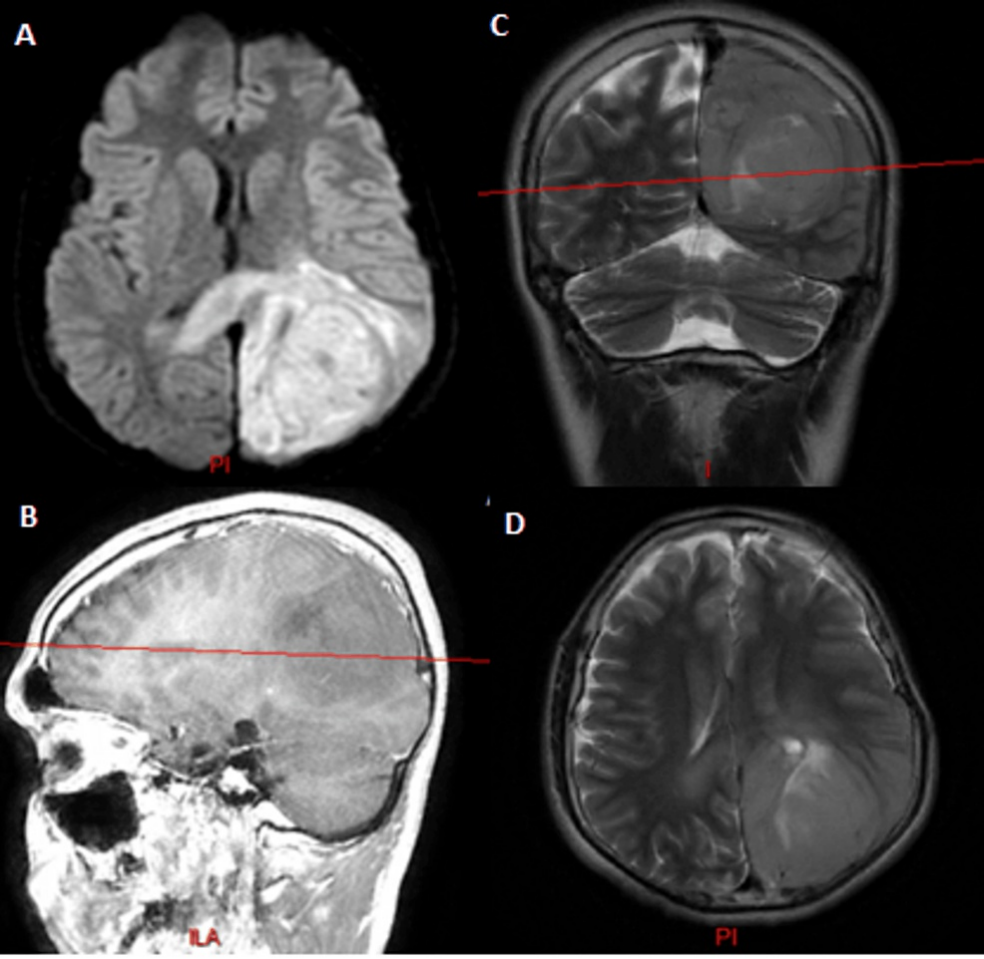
Cranial MRI showing the second lesion developed eight months after left temporal lobectomy (A) Diffusion-weighted image; (B) T1 sequence sagittal view with contrast; (C) T2 sequence coronal view; (D) T2 sequence axial view PI, postero-inferior; I, inferior; ILA, infero-lateral aspect Image courtesy: Al Zahraa Hospital University Medical Center (ZHUMC) Radiology Department
